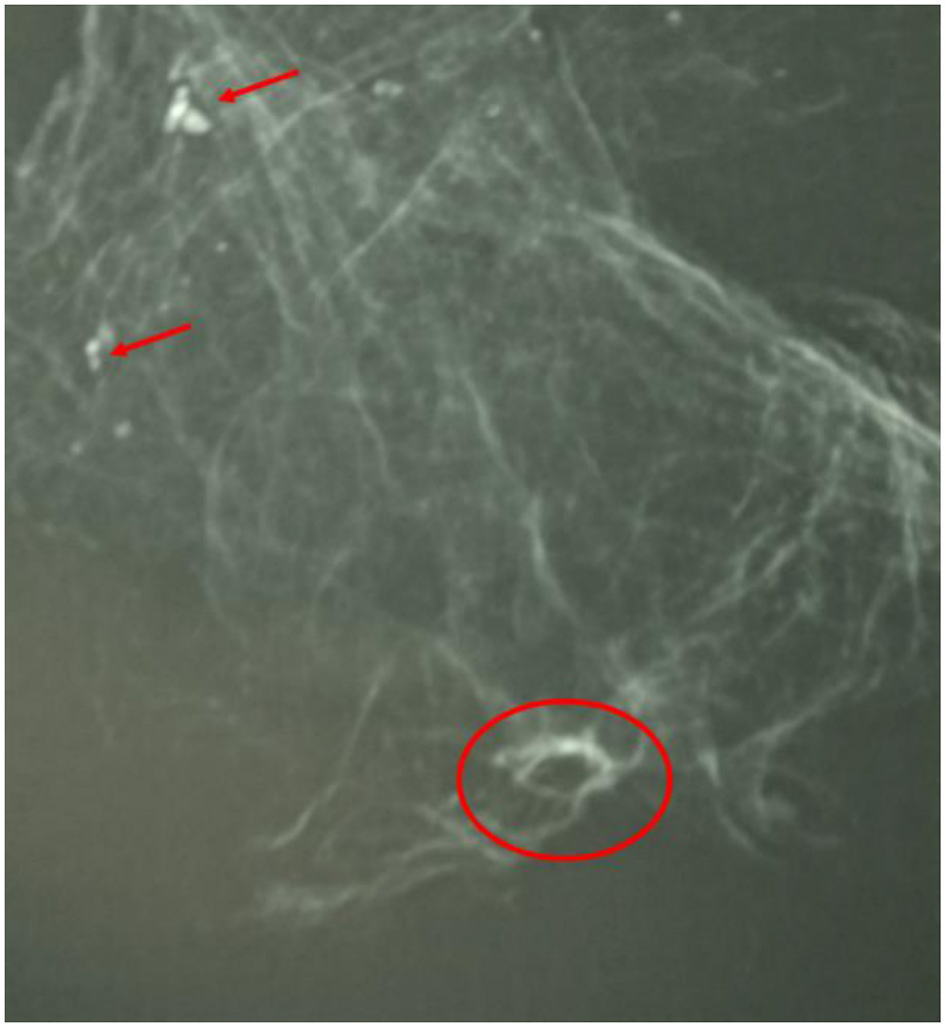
Contact X-ray of the removed right lung. This confirmed the focal calcifications (red arrows) and the cyst with capsular margins (red circle) (image: A. Nerlich).
